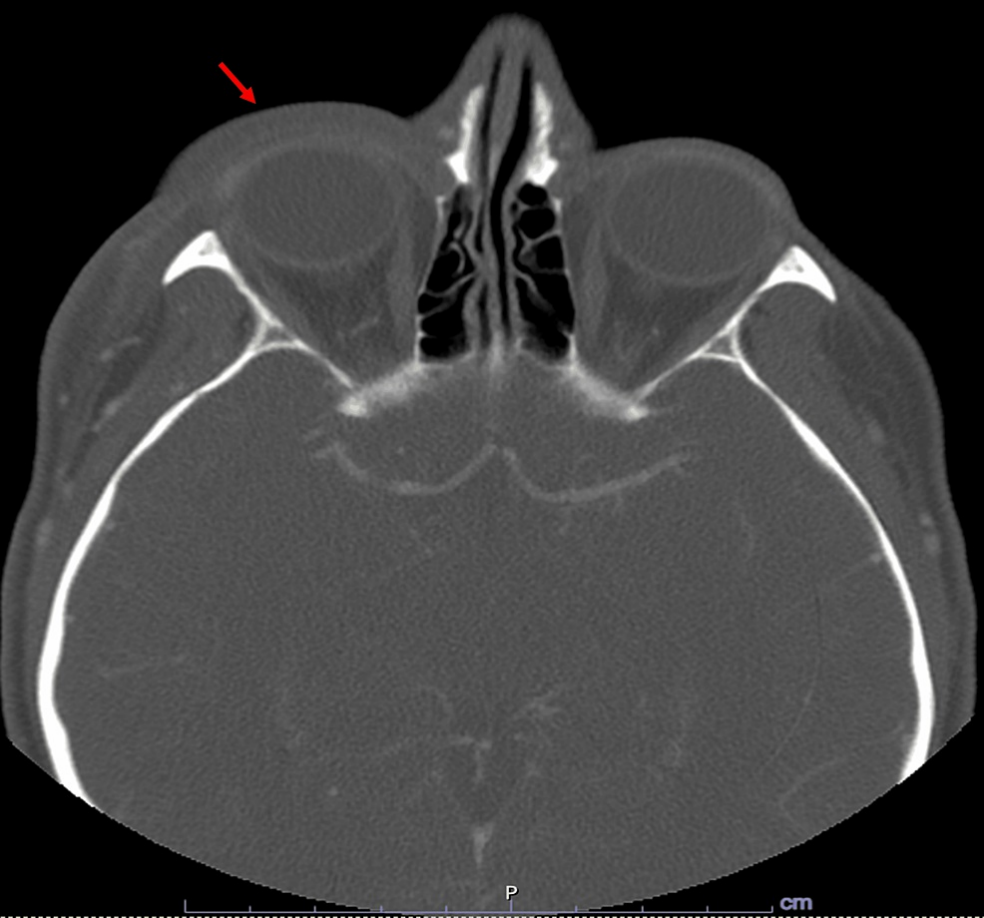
Axial contrast-enhanced CT of orbits and sella turcica showing right periorbital and preseptal soft tissue swelling (red arrow) CT: computed tomography
